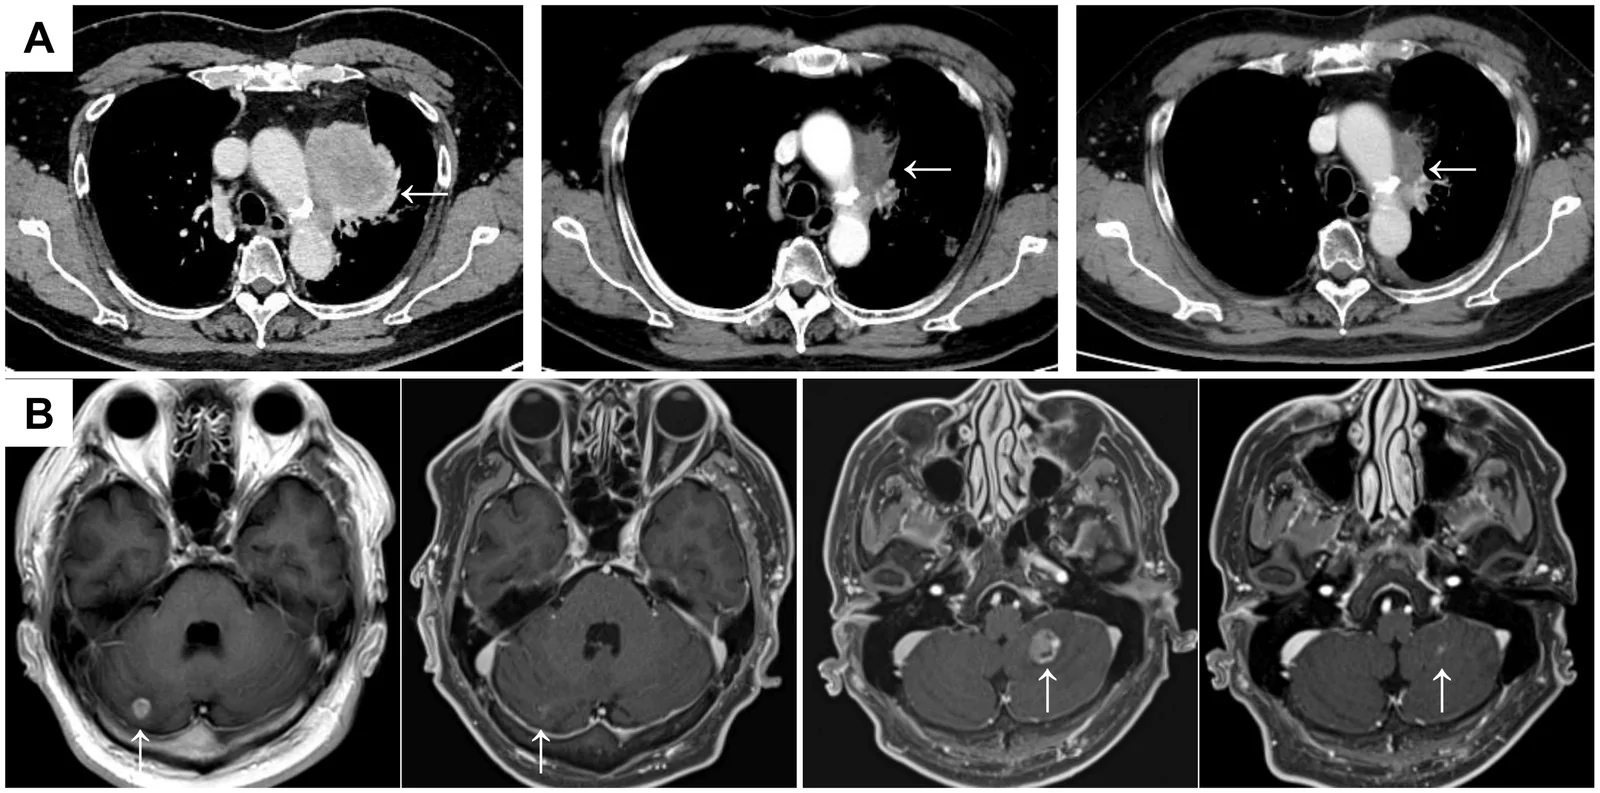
Radiological course of Case 1. (A) Computed tomography images of the para-aortic lymph node lesion before (left) and after (middle) radiotherapy. Radiotherapy was performed before tarlatamab initiation. The para-aortic lymph node lesion during tarlatamab treatment (right) shows sustained local control. (B) Magnetic resonance images showing the right cerebellar metastasis before the first course of stereotactic radiotherapy (far left) and its regression after treatment, before tarlatamab initiation (center left). A newly developed left cerebellar metastasis during tarlatamab treatment is shown before the second course of stereotactic radiotherapy (center right) and after its complete radiographic resolution following treatment (far right). White arrows indicate the para-aortic lymph node lesion and cerebellar metastases.
